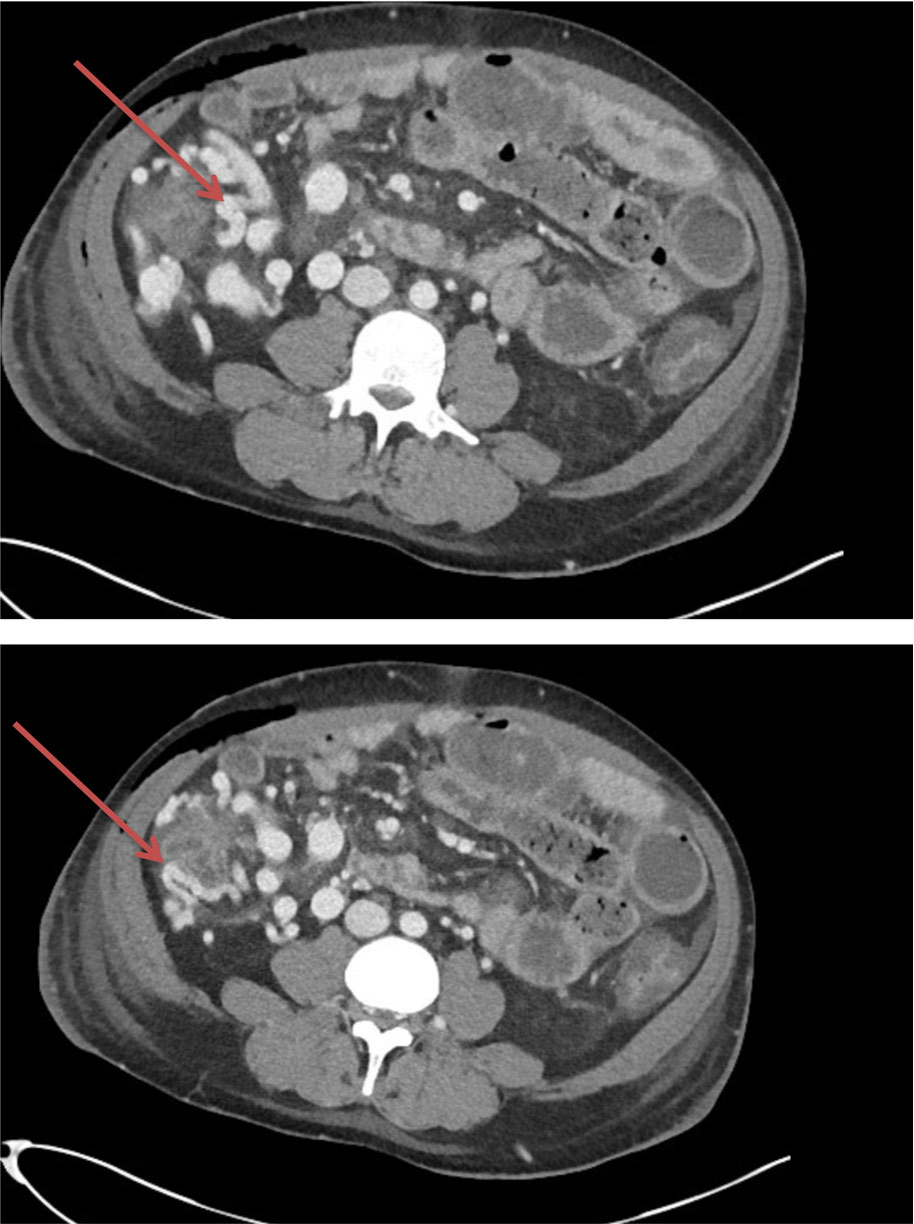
Computed tomography mesenteric angiogram showing cecal varices.
